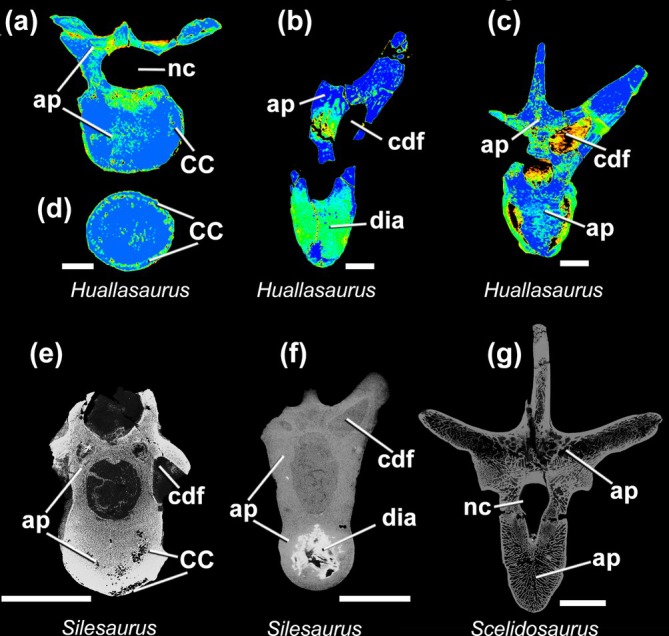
CT scan slices comparison between the vertebrae of ornithischians and *Silesaurus*. The hadrosaur *Huallasaurus* MACN‐Pv RN826.a (a,d); MACN‐Pv RN826.b (b); MACN‐Pv RN 02.c (c). *Silesaurus* ZPAL Ab III 1299 (e); ZPAL Ab III 423/6 (f) (Butler et al., 2012). (g) The thyreophoran *Scelidosaurus* NHMUK R1111 (Butler et al., 2012). Cervical vertebrae in (a–c). Dorsal vertebrae in (b,c,f,g). All in transverse view. ap, apneumatic bone; cc, circumferential chambers; cdf, centrodiapophyseal fossa; dia, diagenetic artifacts; nc, neural canal. Scale bar in a–d,g = 20 mm; in e,f = 10 mm.
